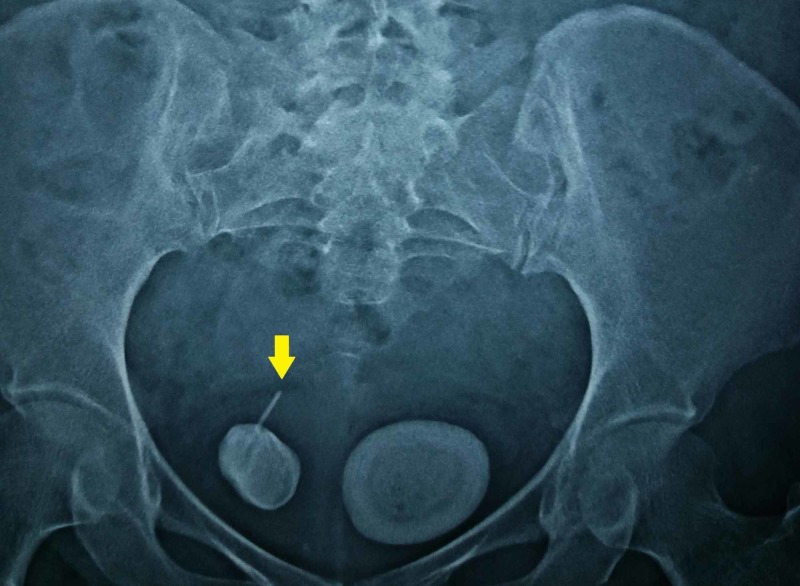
X-ray image of the kidney, ureter, and bladder region showing the presence of two vesical calculi with a linear radio-opaque extension suspicious of foreign body (yellow arrow)
